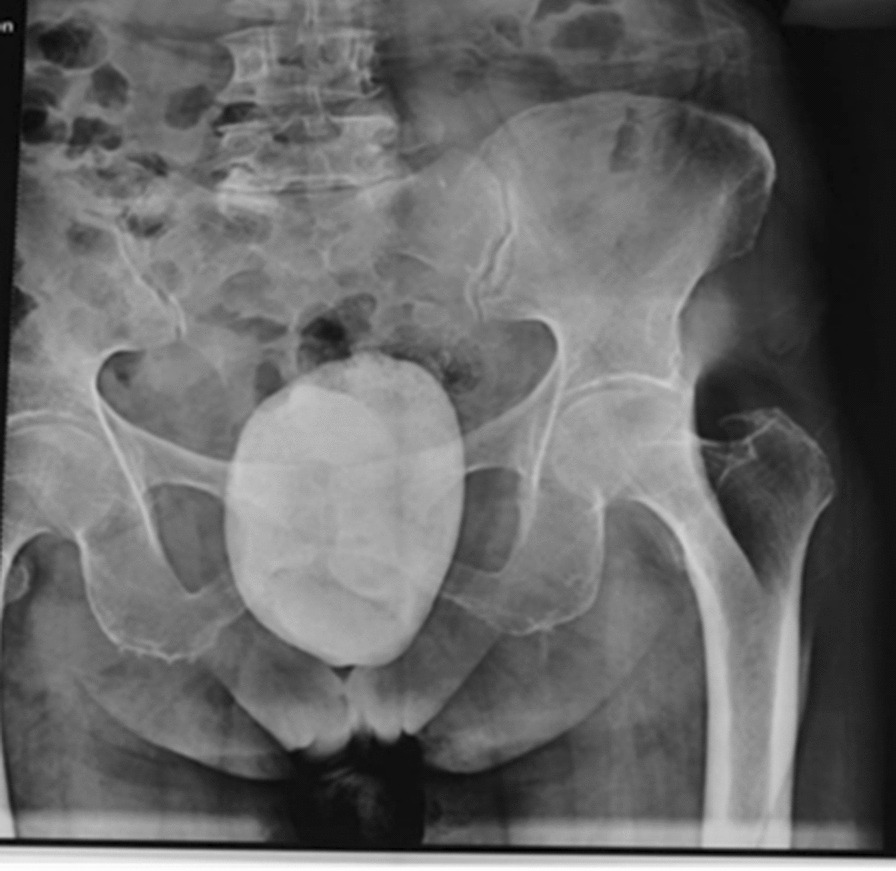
Anteroposterior pelvic radiograph showing a radio-opaque ovoid mass in the lower pelvis, suggestive of a bladder stone
